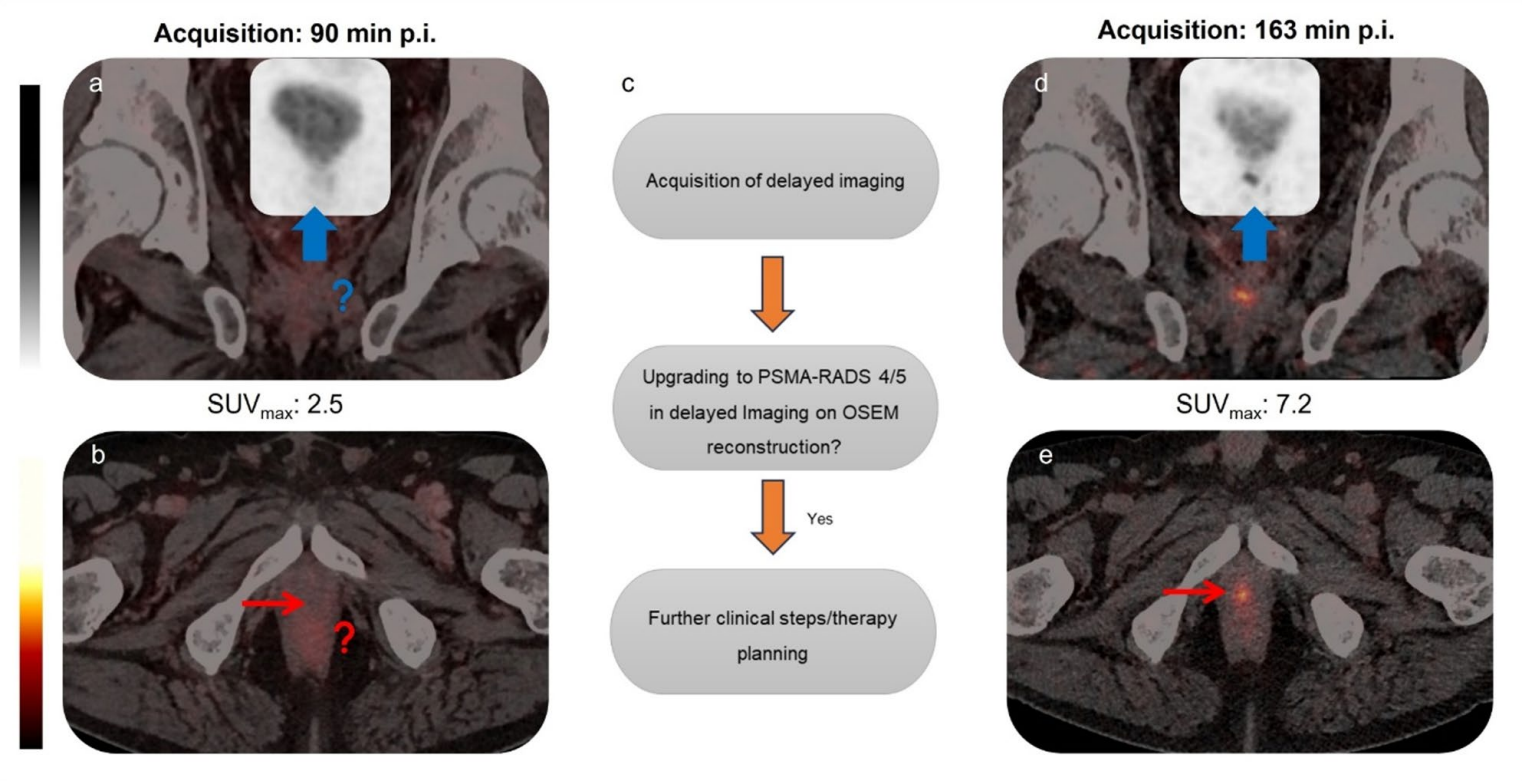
Delayed scan of a 64-years-old patient with suspected local relapse (a & b) underlined its utility by upgrading (d & e) the reporting certainty by the reader in terms of PSMA-RADS V2 (c)
